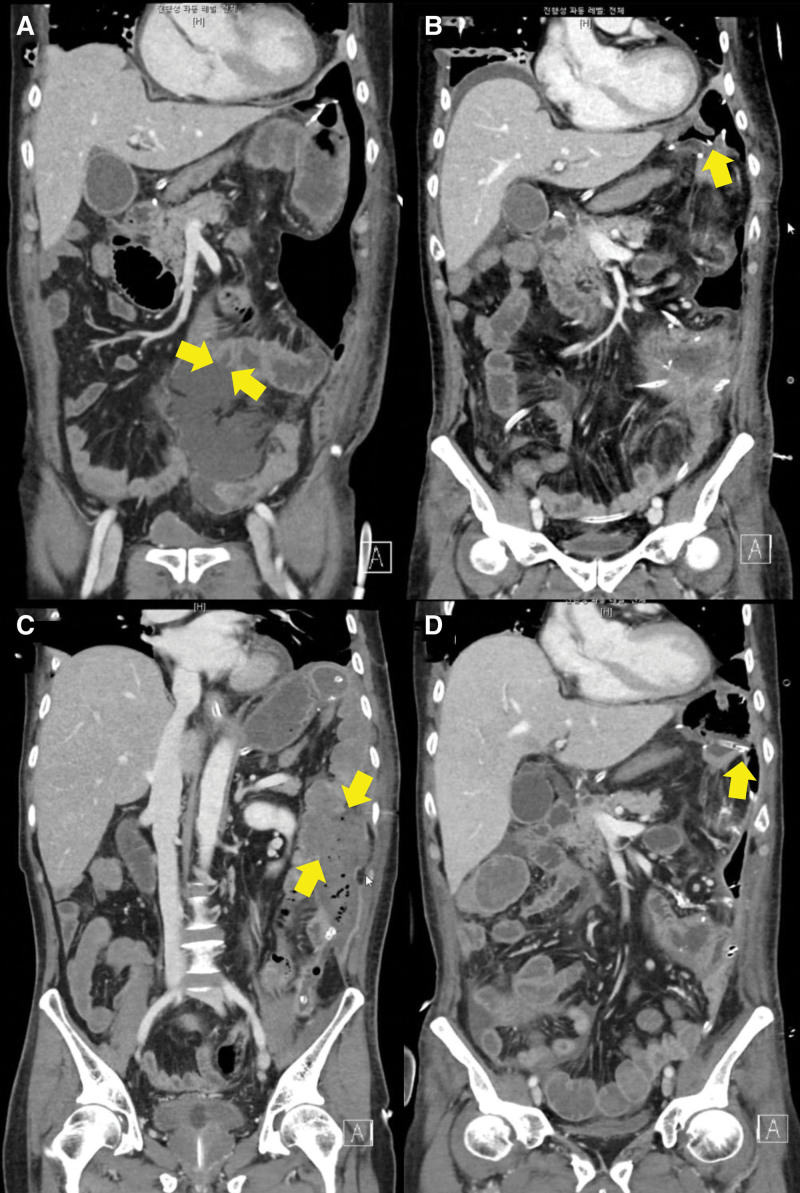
Serial abdominal CT images demonstrating progressive gastrointestinal complications in Case 2. (A) Coronal CT image on March 5, 2025 shows a 1-cm wall defect in the mid-descending colon with adjacent fluid collection in the left abdomen (yellow arrows), and an additional 0.8-cm wall defect abutting the small bowel near the jejunojejunostomy site. (B) On March 7, 2025, a small defect is noted at the gastrojejunal anastomosis, accompanied by peri-gastric free air (yellow arrow). (C) CT on March 22, 2025 reveals interval progression with a large (4.2 cm) wall defect of the mid-descending colon and a dehisced (approximately 4 cm) gastrojejunal anastomosis (yellow arrows). (D) Follow-up CT, on March 30, 2025, shows probable redevelopment of a approximately 4 cm defect at the gastrojejunostomy site (yellow arrow), and a questionable small dehiscence at the previously repaired descending colon.
